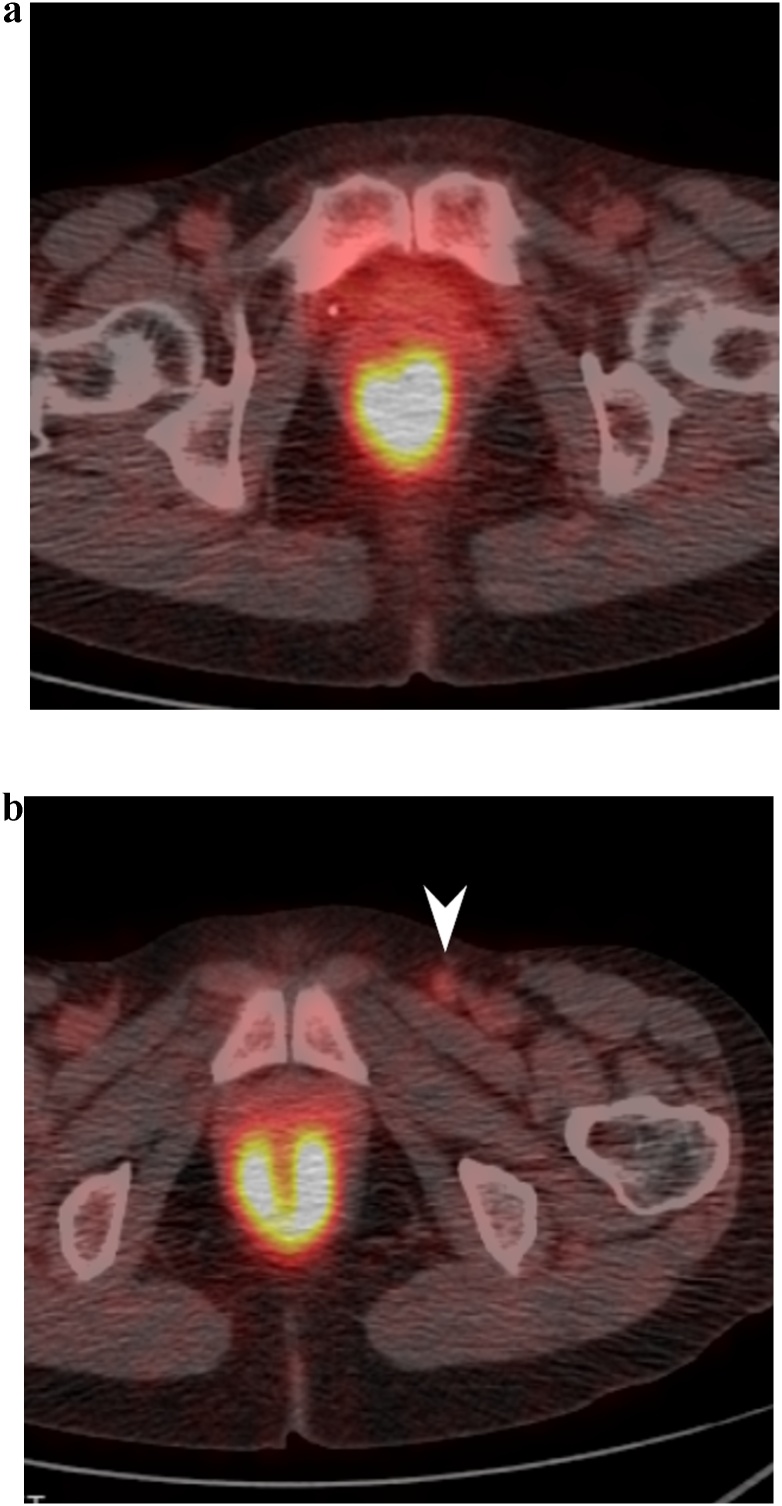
PET findings. a, b: PET revealed an accumulation of FDG in the anal canal mass and left inguinal lymph nodes (white arrow head) which were detected by CT.
